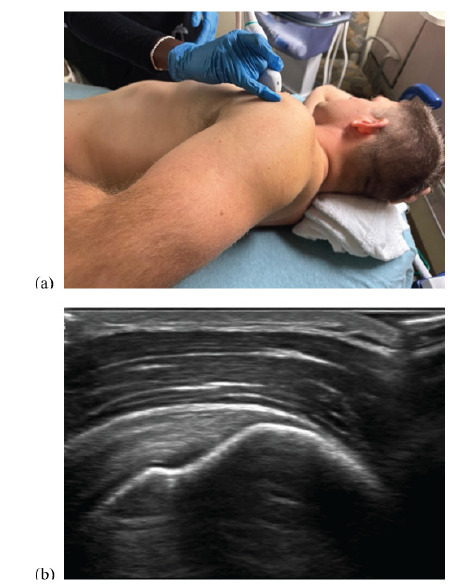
(a) Positioning of the ultrasound probe to obtain the long-axis view of the supraspinatus tendon using the supra-short protocol; and (b) sonographic long-axis view of a normal supraspinatus tendon.
